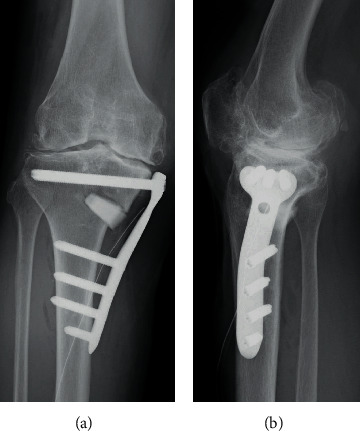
Postoperative radiography shows that tibial condyle valgus osteotomy with an open distance of 11 mm was performed and fixed with a metal plate. (Anteroposterior view (a) and lateral view (b)).
